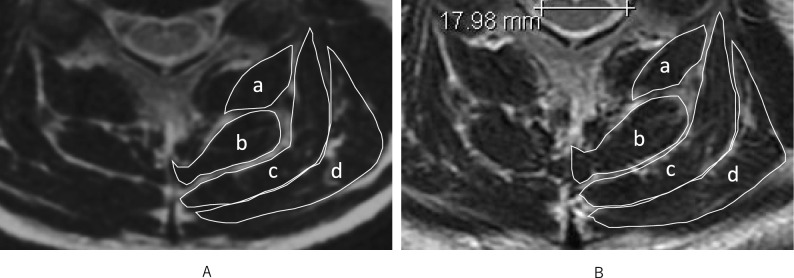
Axial T2-weighted MRI images at the C4/5 level from the same participant. (A) Baseline MRI. (B) 20-year follow-up MRI. The cross-sectional areas of the posterior extensor muscles—(a) Multifidus, (b) Semispinalis cervicis, (c) Semispinalis capitis, and (d) Splenius capitis—were segmented using ImageJ software. ROIs were manually traced within visible fascia boundaries.
